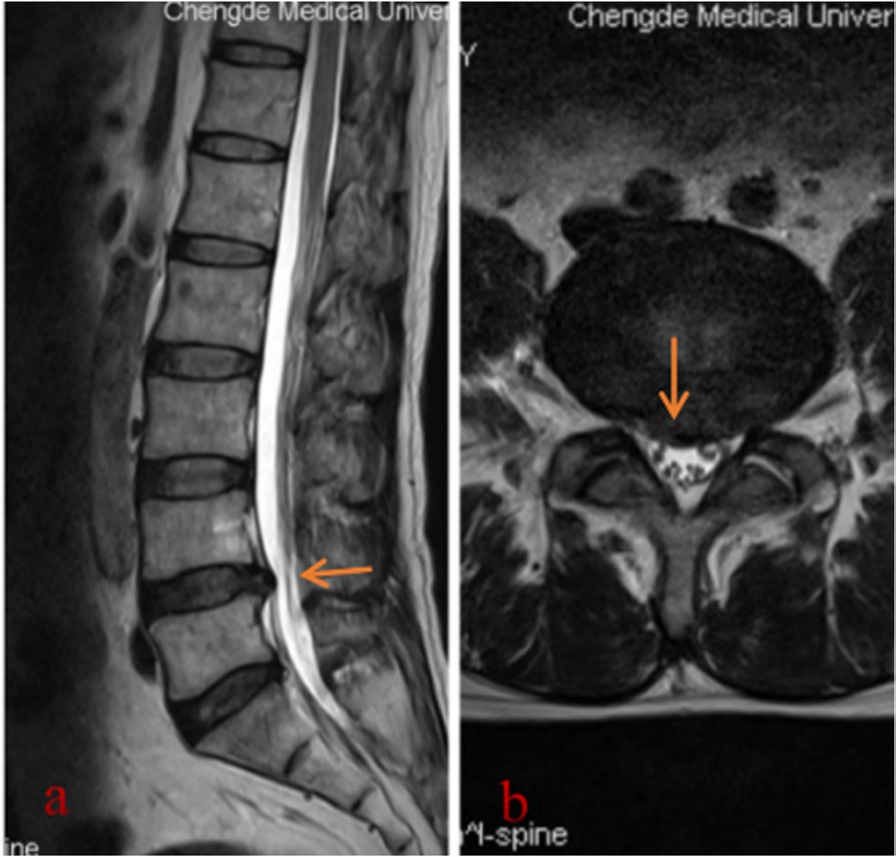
Lumbar MRI in case 1. a Sagittal MRI shows L4-5 intervertebral disc herniation and compression of the dural sac (arrow). b Axial MRI shows lumbar disc herniation and compression of the L5 nerve root (arrow)
